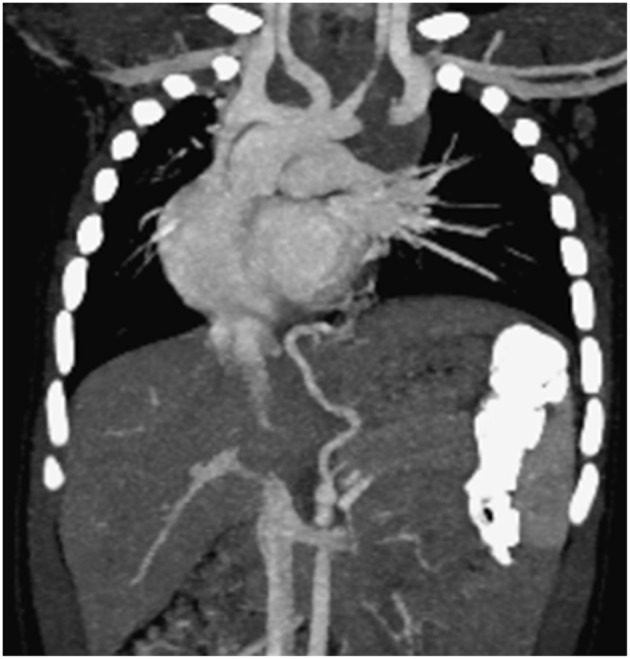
Computed tomography scan documenting two aberrant vessels originating from the tripod celiac artery that lead to the left lower lobe representing intralobar pulmonary sequestration (coronal view).
